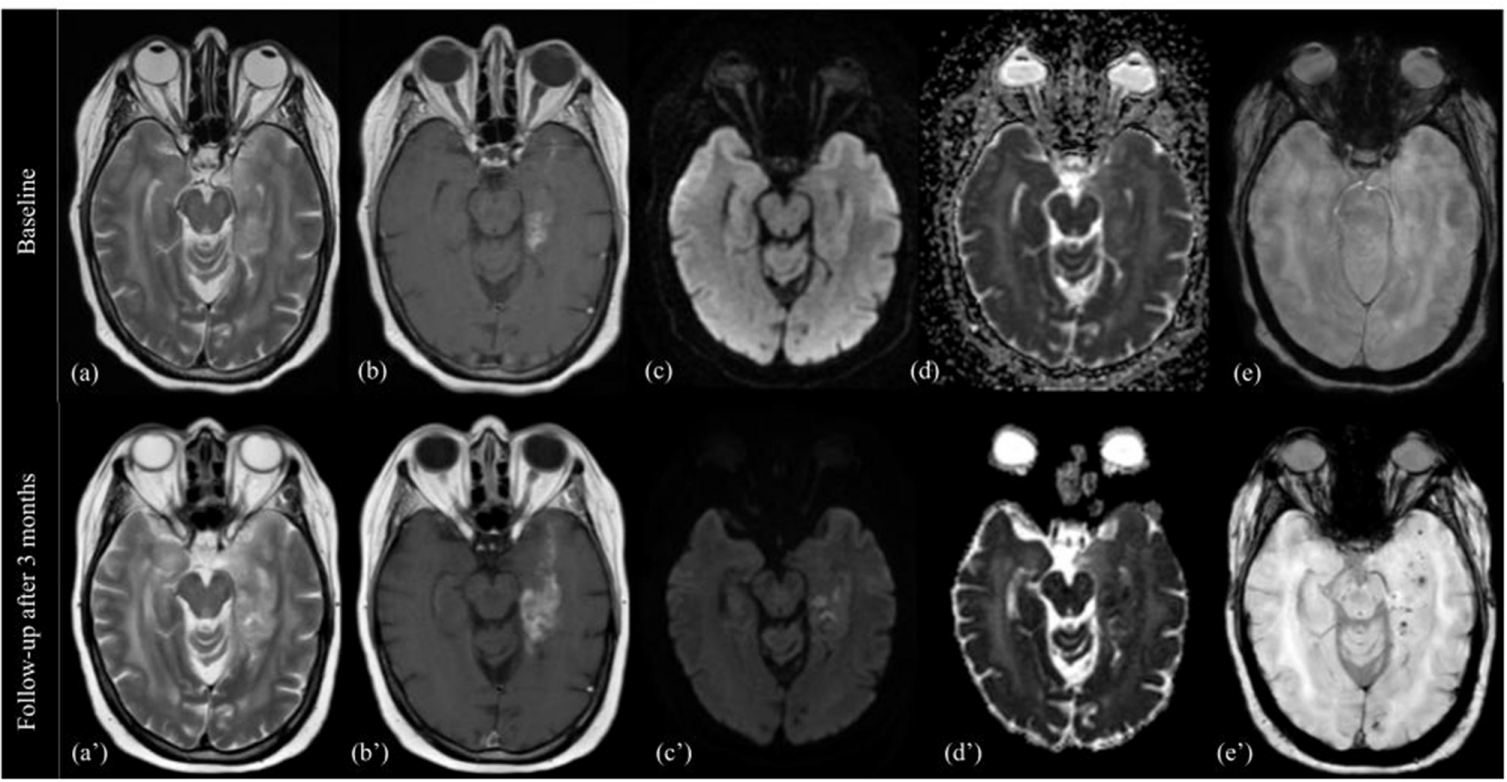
Pattern A. MRI changes in recurrent high grade glioma under regorafenib, pattern A. MRI scans performed at baseline (top image) and 3 months after first administration of REG therapy (bottom image). T2-weighted images (a,a'), T1-weighted images with gadolinium (b,b'), DWI images (c,c'), apparent diffusion coefficient (d,d'), and SWI images (e,e') are shown from left to right. The 3-month follow-up showed increase in size, more than 25%, of enhancing tumor in the left temporo-mesial area, and progressive extension into the ipsilateral parahippocampal gyrus. T2 signal intensity was similar to the previous MR exam, but contained focal hyperintensities on DWI (corresponding to low ADC values), and multiple black dots on SWI.
